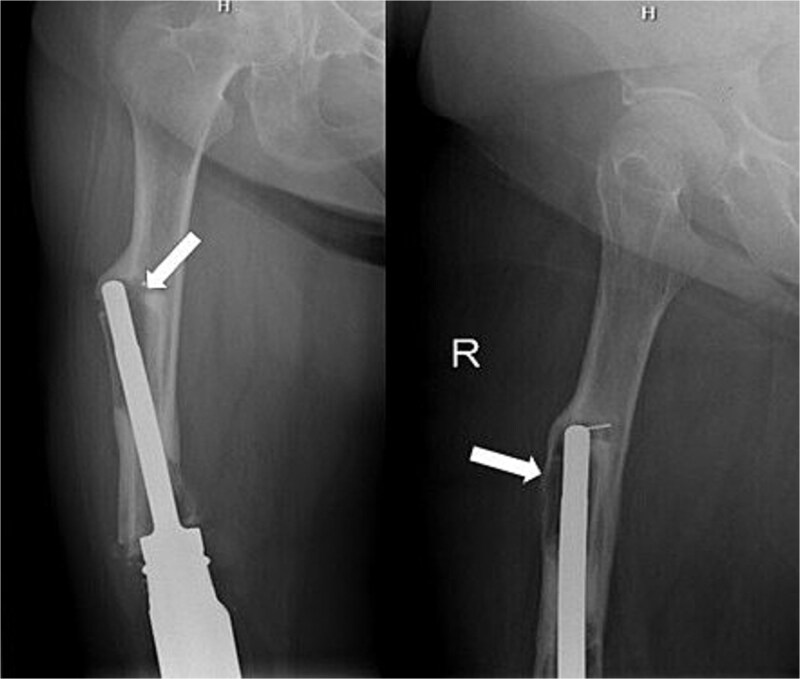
Two-view radiographs of the femur demonstrating radiographic signs of loosening of the cemented intramedullary stem component.
